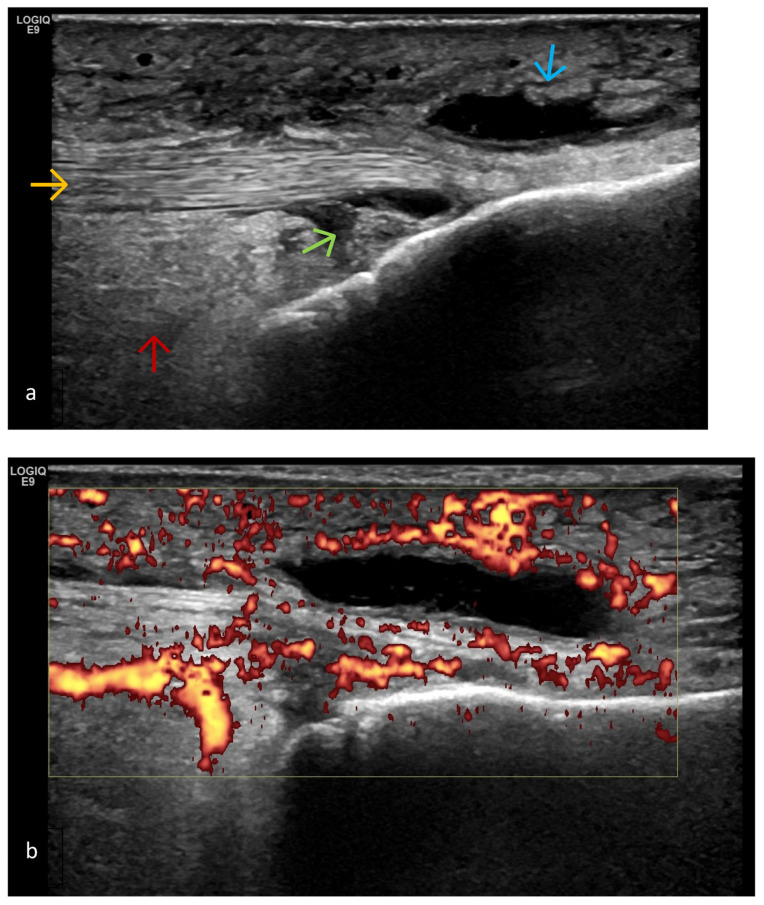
(a) Longitudinal infrapatellar US scan of a patient with knee OA—B-mode. The patellar tendon (orange arrow) is well traced distal to its insertion on the tibia. There is superficial (blue arrow) and deep (green arrow) infrapatellar bursitis with a mixed component—both proliferative with synovitis and exudative with exudate. Hoffa’s fat pad synovitis is also clearly visible (red arrow). (b) Power Doppler modality reveals highly active disease, grade 3.
